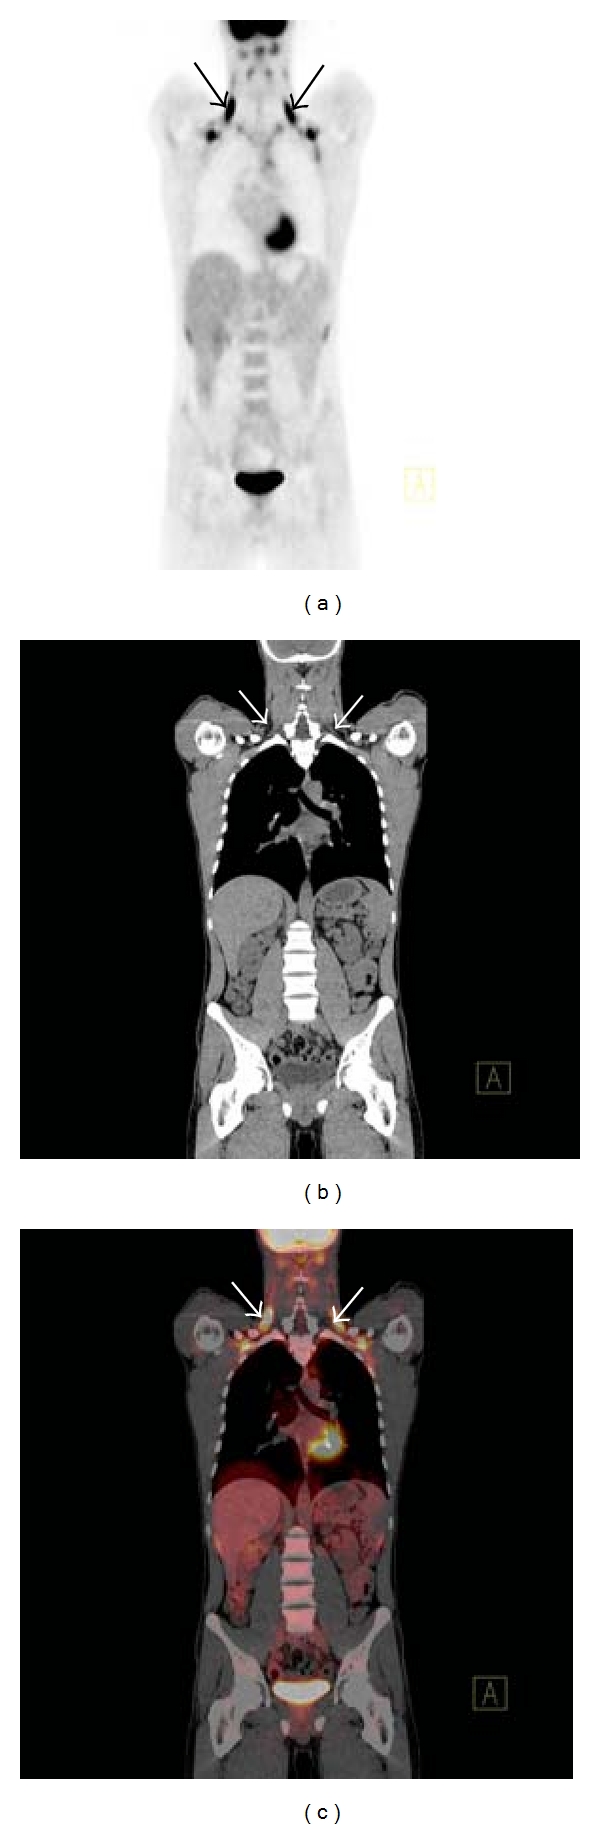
Coronal PET maximum intensity projection (MIP) image (a) displaying foci of increased radiotracer uptake bilaterally in the neck in a patient with treated DLBSL. The corresponding coronal CT (b) and fused FDG-PET/CT (c) demonstrate no abnormality and the areas with increased FDG uptake correspond to regions of fat density (arrows). This is the characteristic appearance of hypermetabolic brown fat on FDG-PET/CT.
